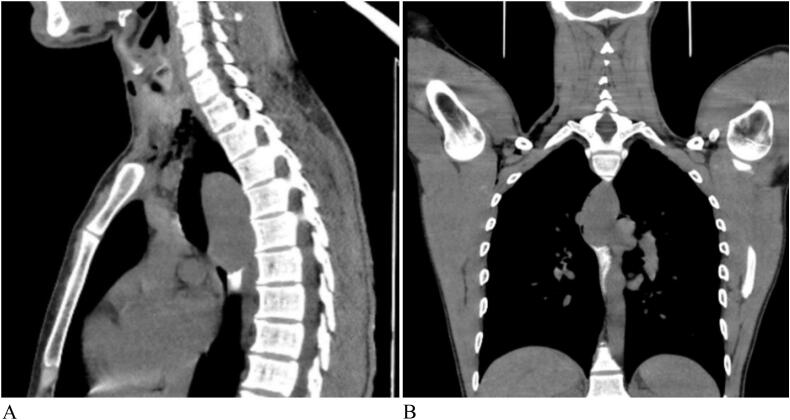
CT image showing a 3.4 cm × 2.5 cm × 5.3 cm soft tissue mass confined to the esophageal wall, with no evidence of invasion into surrounding structures, suggesting a submucosal origin.
